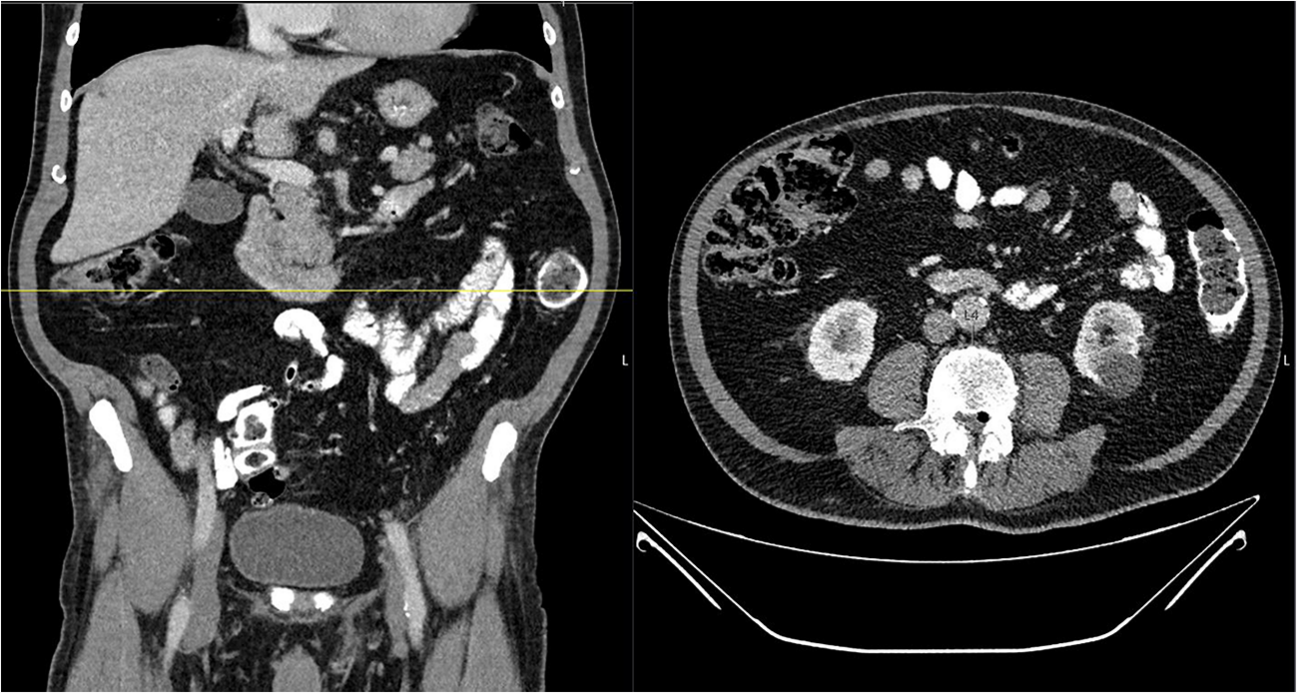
The abdominal CT scan excludes the suspected diagnosis of an abdominal wall herniation
